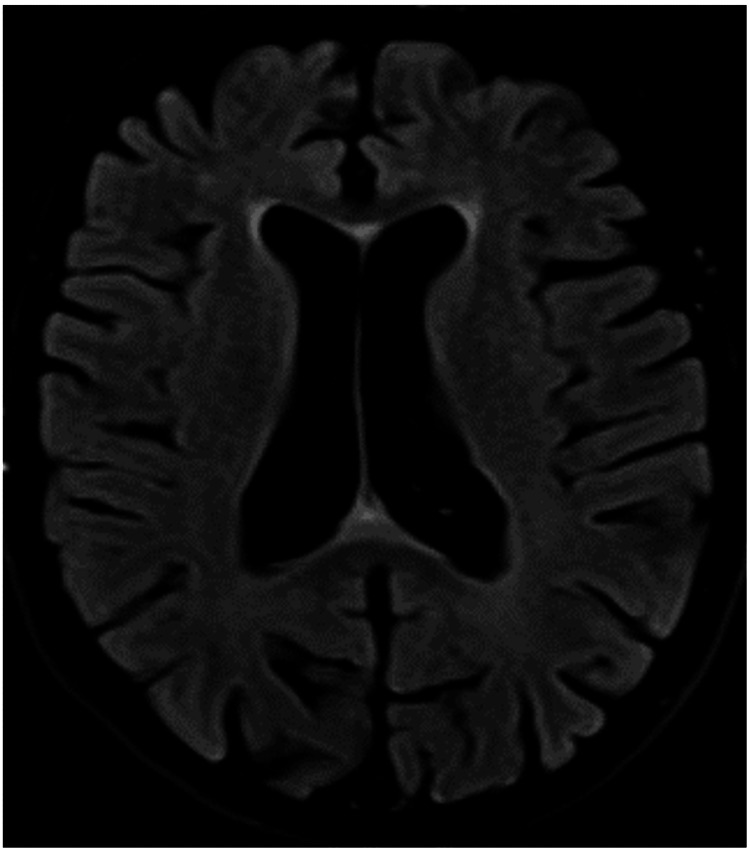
Brain MRI findings: Axial FLAIR image obtained three months after clinical onset. MRI, magnetic resonance imaging; FLAIR, fluid-attenuated inversion recovery
